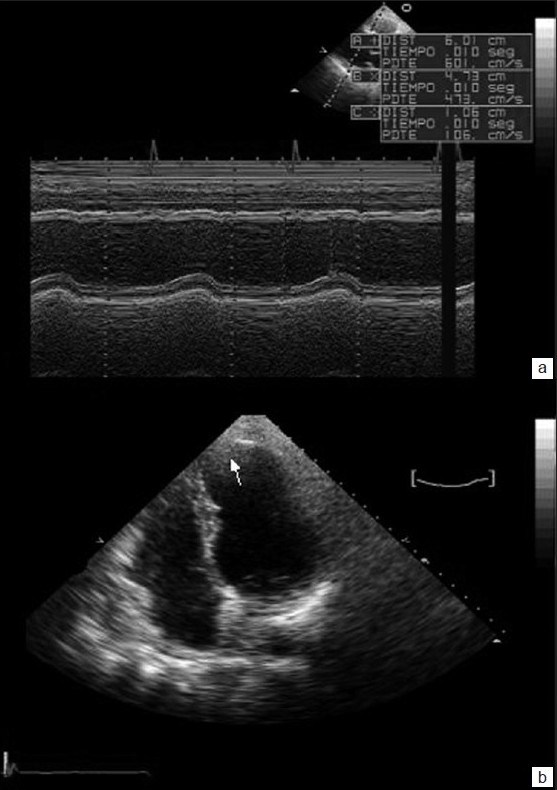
(a) M-mode obtained from the left paraesternal long axis view reveals no interventricular septum thickness and normal contraction of the posterior wall; (b) apical four-chamber 2-D echocardiogram with apical aneurysm (arrow)
